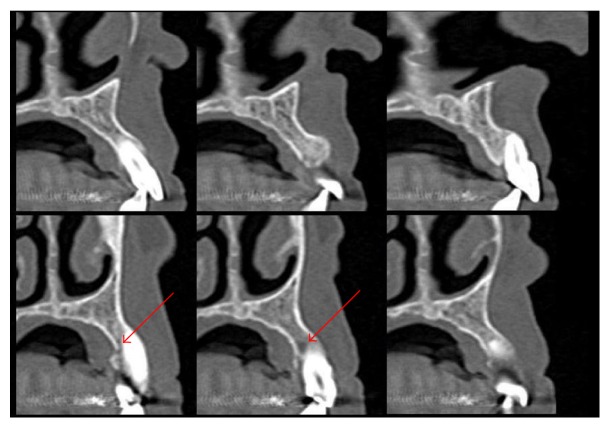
Preoperative CT. The red arrows point to the small depression of the bone surface in correspondence of the tumour (“cupping” or “saucerization”).
